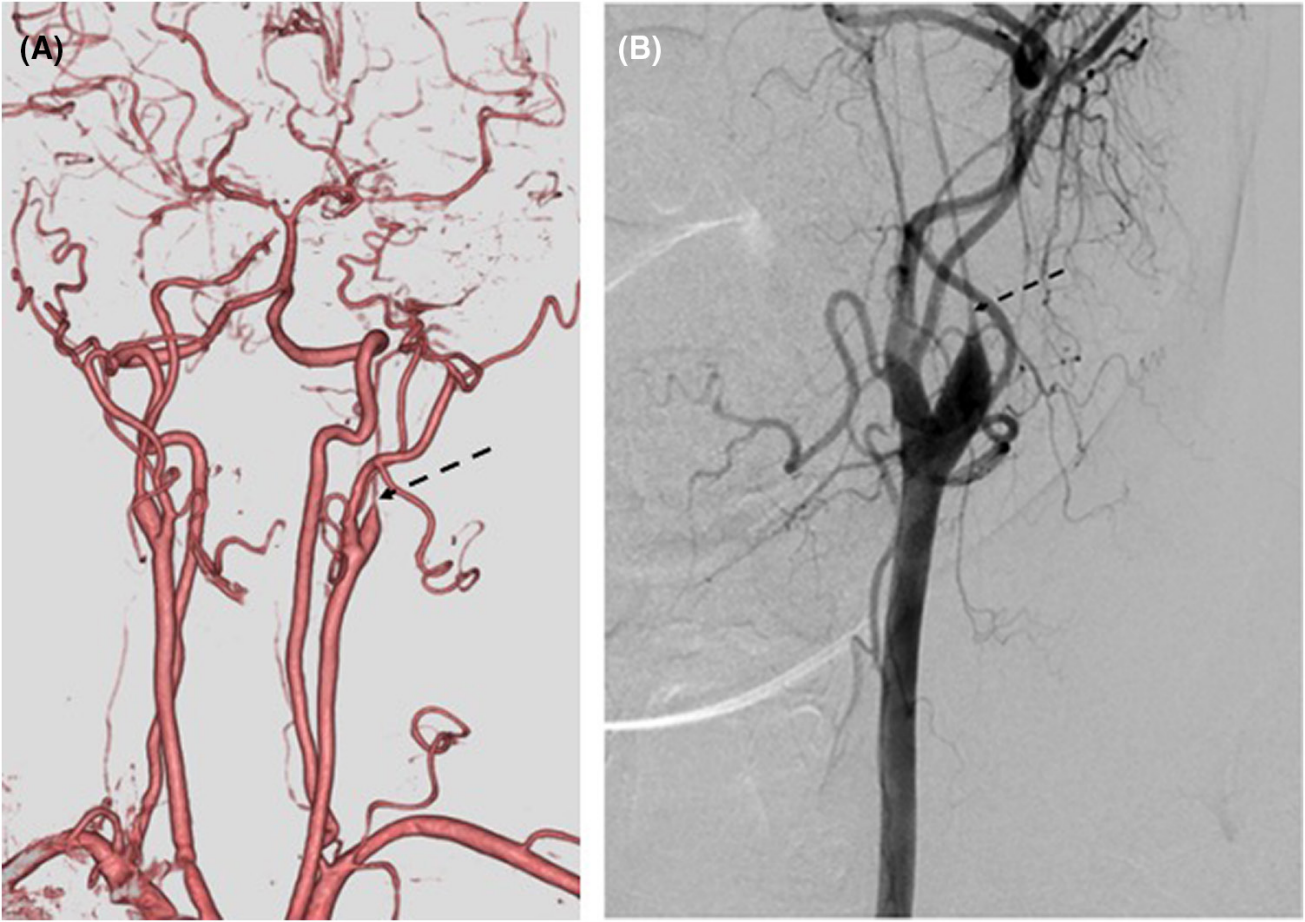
(A) Three‐dimensional head computed tomography indicates severe hypoplasia of the left internal carotid artery (dotted black arrow). (B) Carotid angiography shows a champagne bottle‐neck sign in the proximal portion of the internal carotid artery (dotted black arrow).
